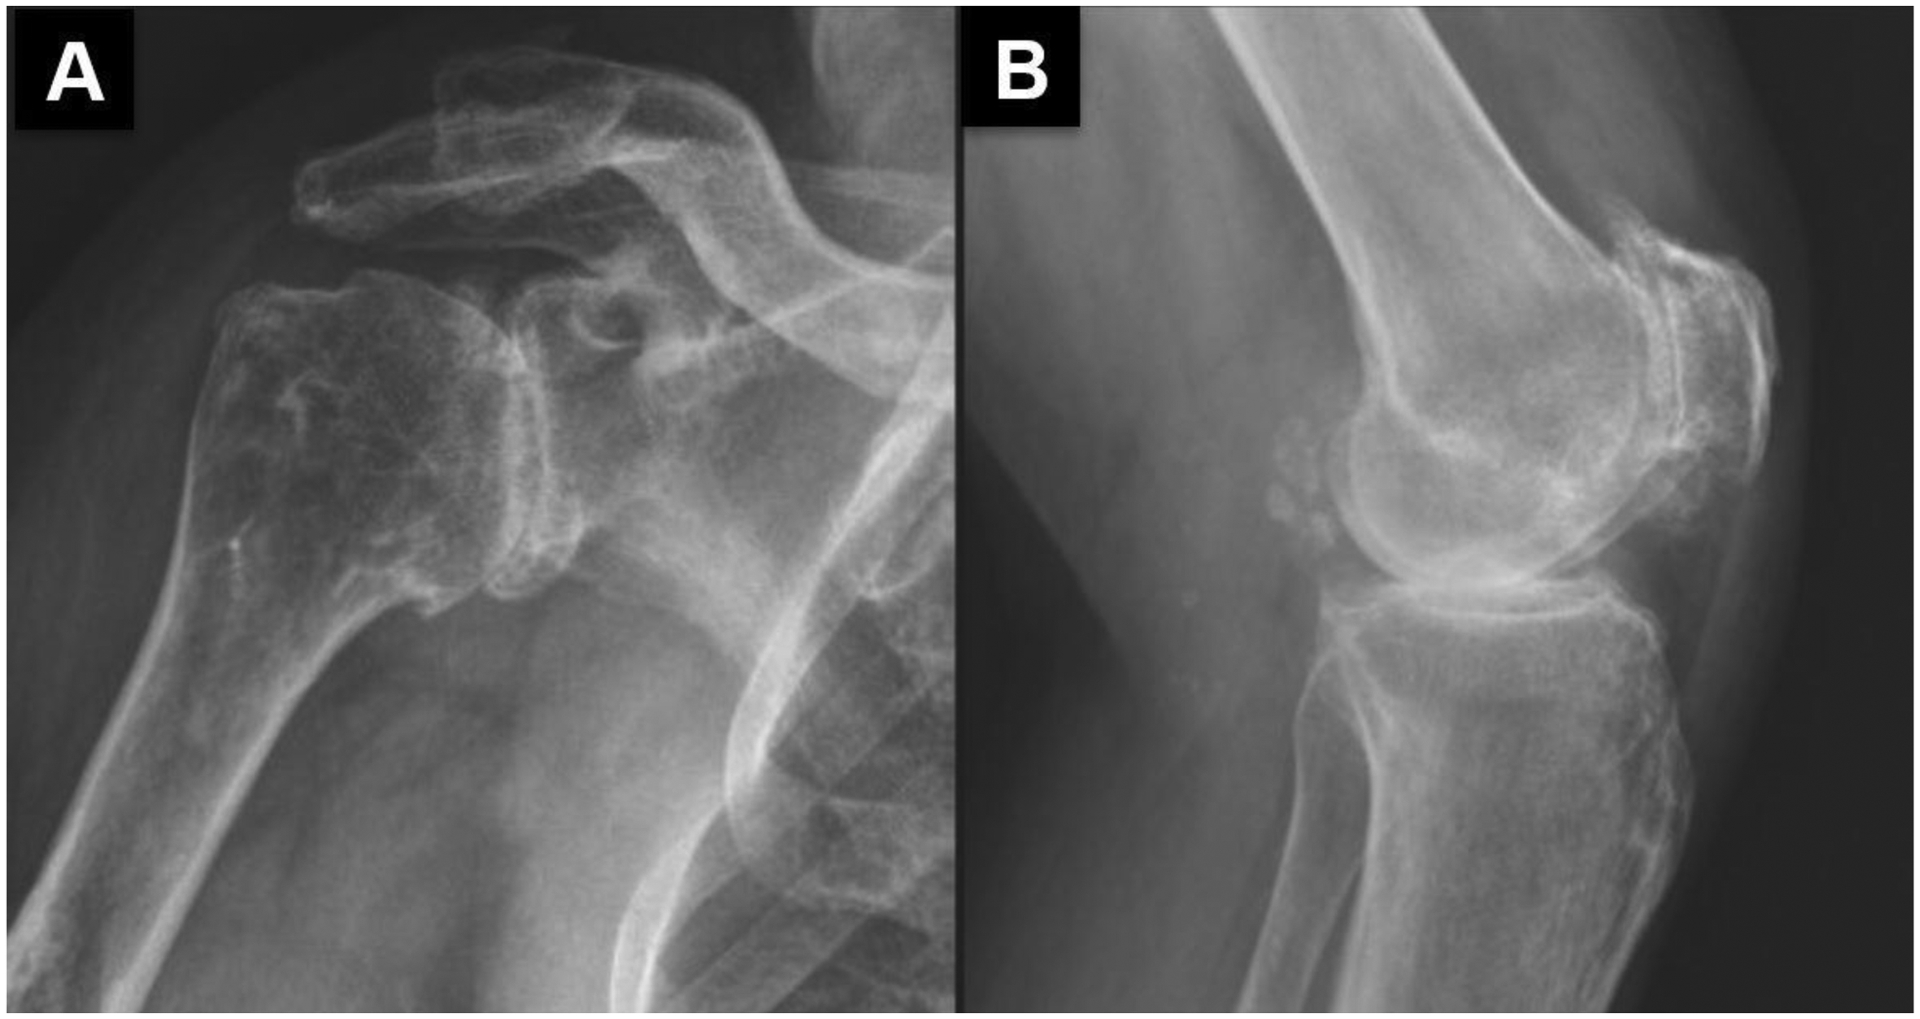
Radiographs of the ochronotic patientś right shoulder (a) and left knee (b) showing severe osteoarthritis, chondrocalcinosis is present in the popliteal bursa. Reproduced with permission [33].
